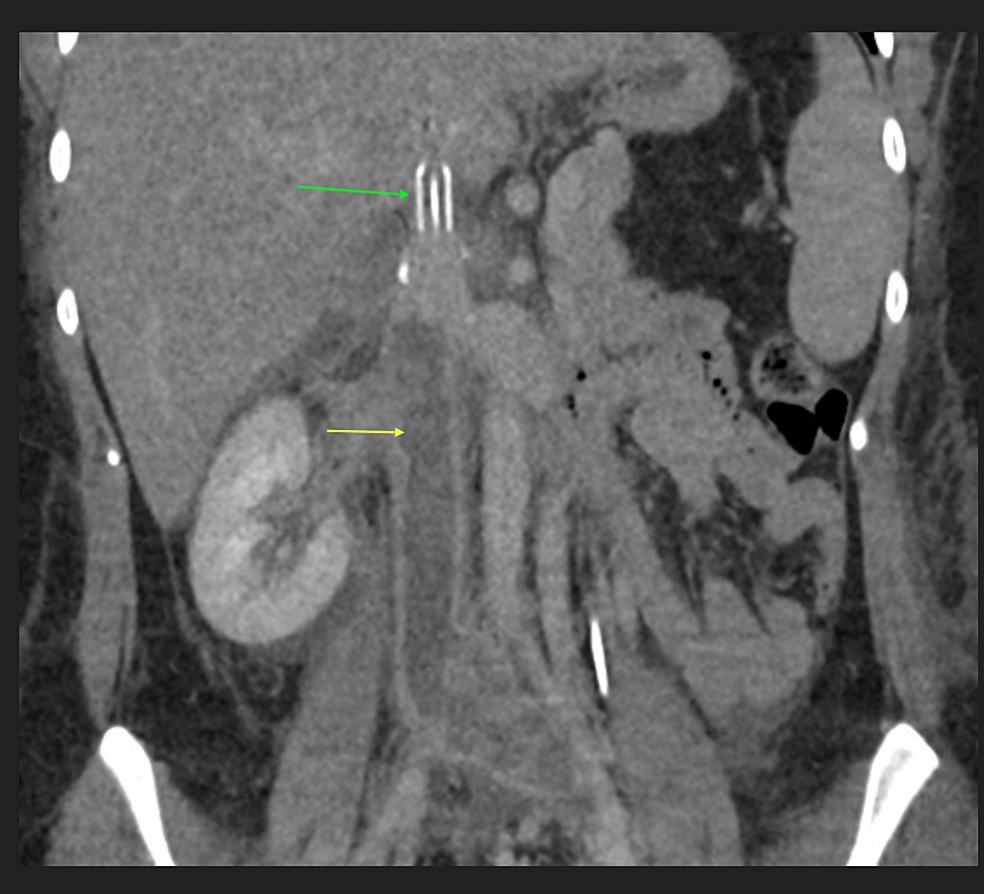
Contrast-enhanced coronal CT image of the abdomen shows re-thrombosis of the IVC after partial thrombectomy and placement of IVC filter (partially visualized, green arrow), the yellow arrow shows the infra-renal IVC.
